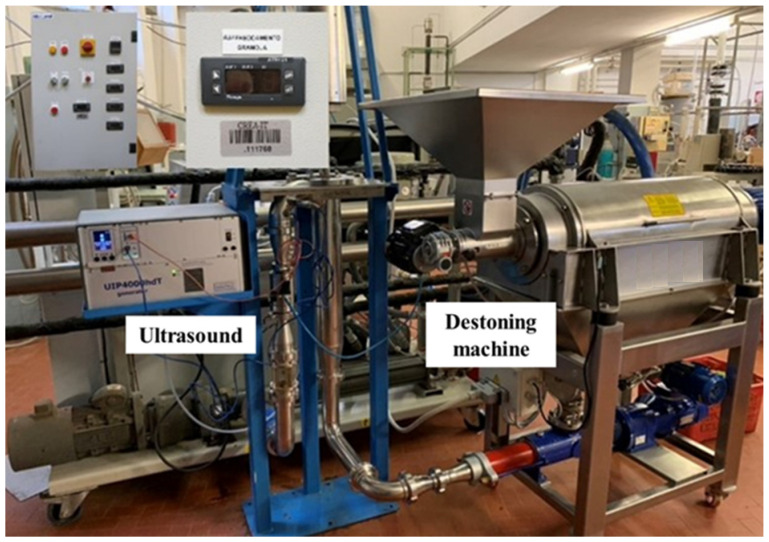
The experimental system consists of the ultrasound and destoning machine, used for the tests at the Department of Agricultural, Food, and Environmental Sciences of the University of Perugia (Italy).
